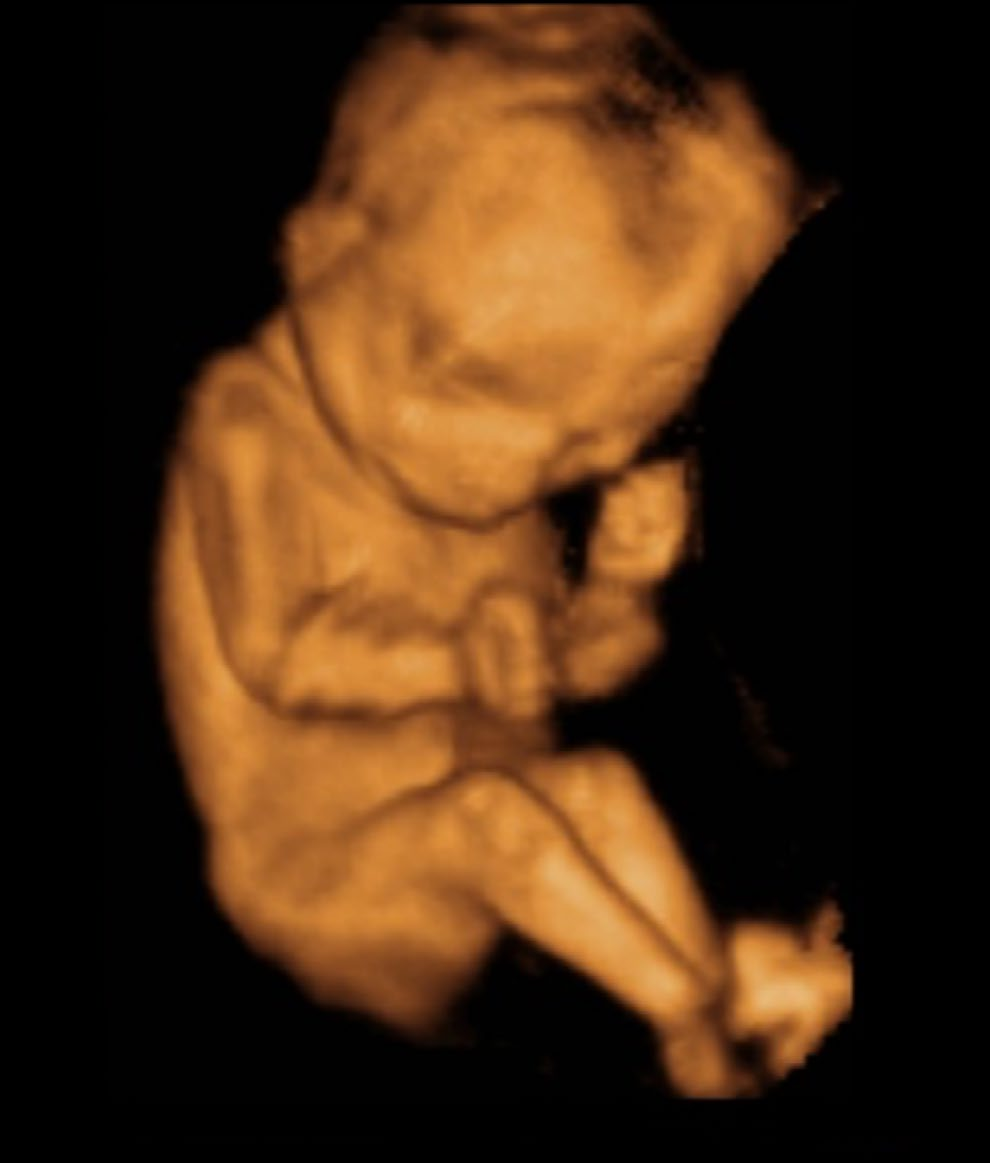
Three‐dimensional ultrasound imaging showing abnormal positions of the upper and lower extremities of a fetus with AMC.
